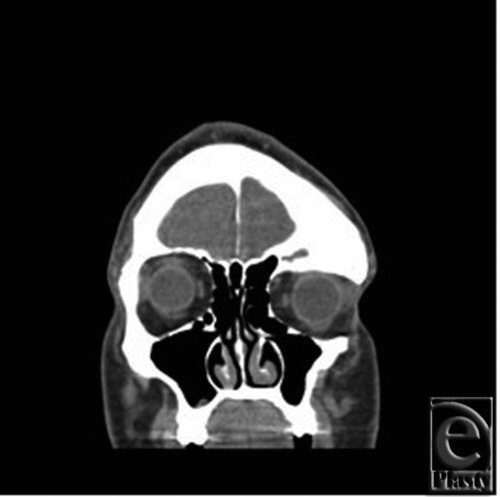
Preoperative coronal computed tomographic image demonstrating dysplastic growth of the left temporal bone with frontal involvement and displacement of orbital contents.
